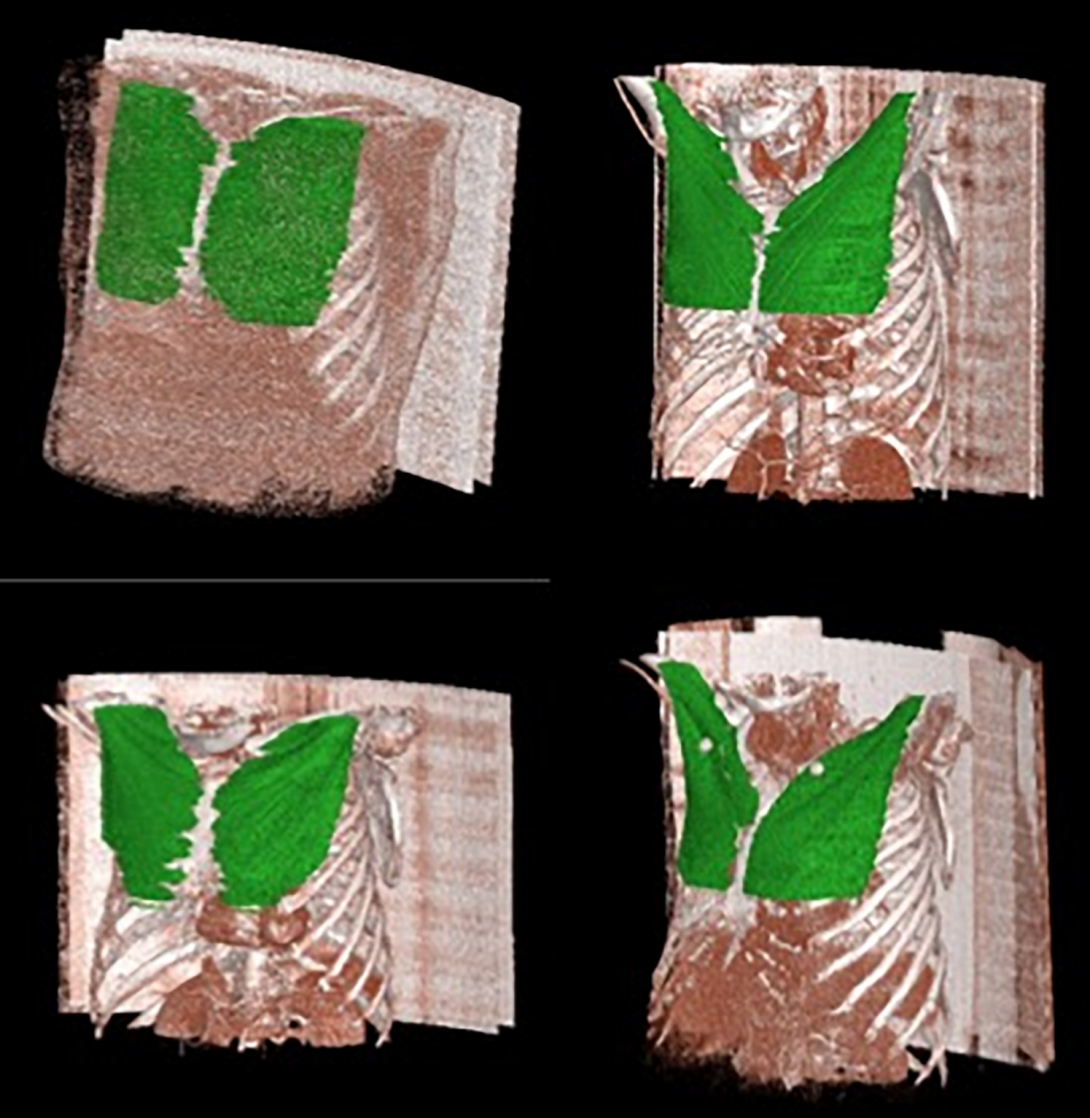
Sample case of a patient CT image with an overlayed label of the pectoralis major muscle.
